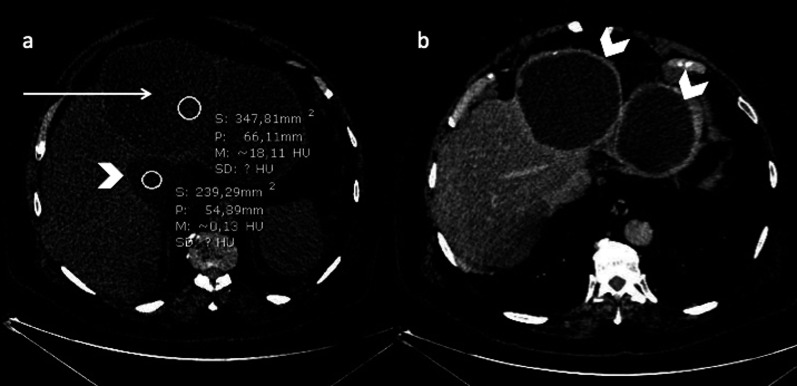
Infected simple hepatic cysts in a 77-year-old male with autosomal dominant polycystic liver disease. a Axial non-enhanced computed tomography shows two cysts under pressure (arrow), slightly more attenuating than the non-infected cysts (arrowhead); b with a thick enhanced wall on the portal venous phase (arrowhead)
